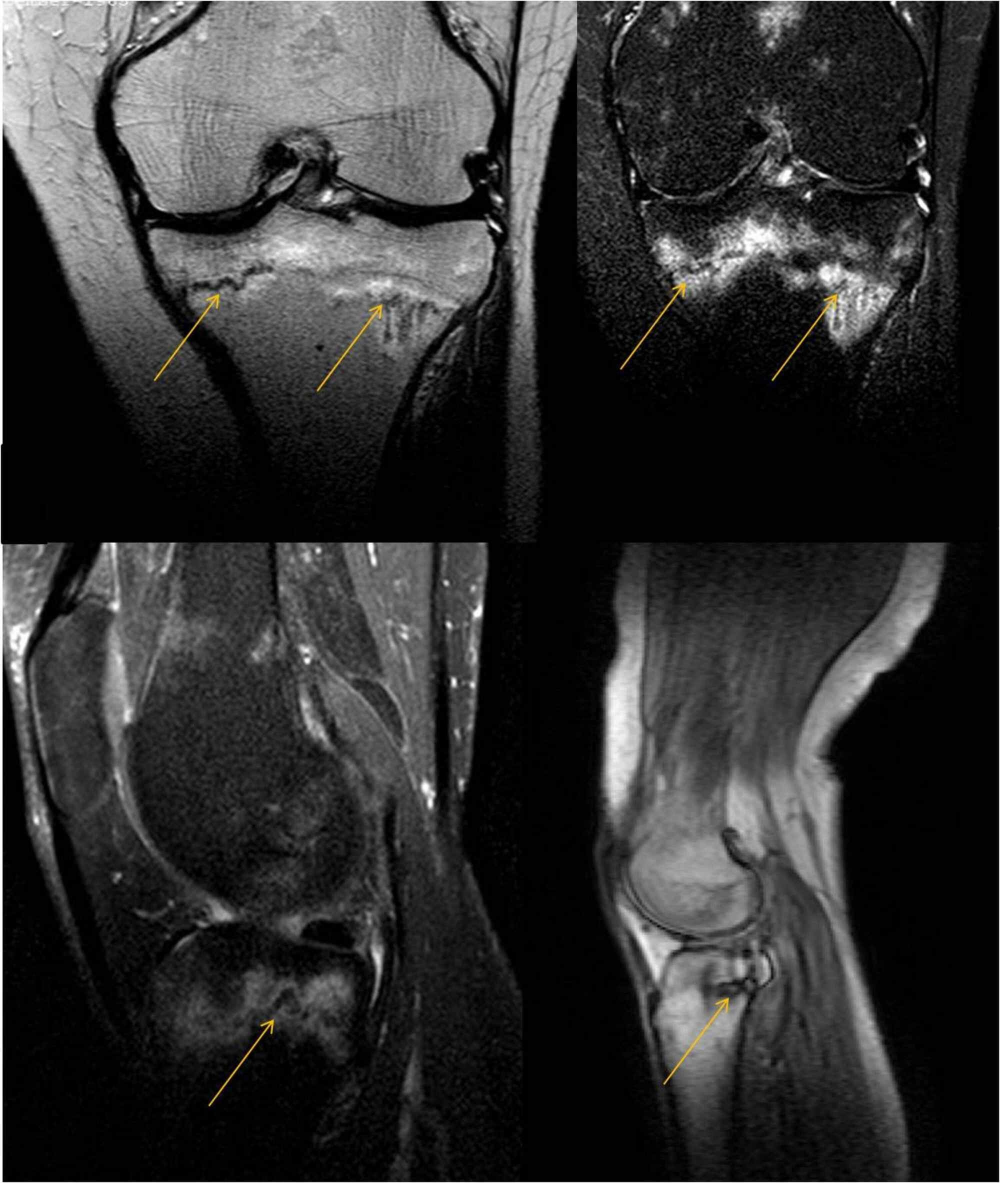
MRI of the left knee. The yellow arrows point to insufficiency fracture lines. Edema is obvious around the fracture lines.
